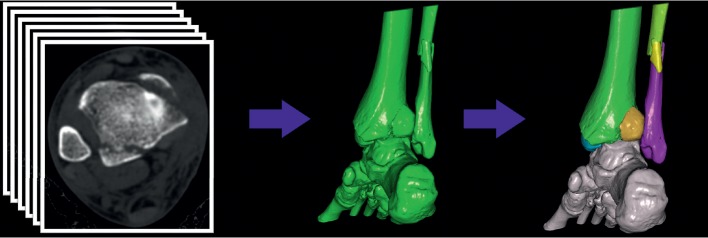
Three-dimensional processing of thin-section axial CT images is shown. Three-dimensional images (surface shaded display) were generated, and all component bones were distinguished by different colors.
